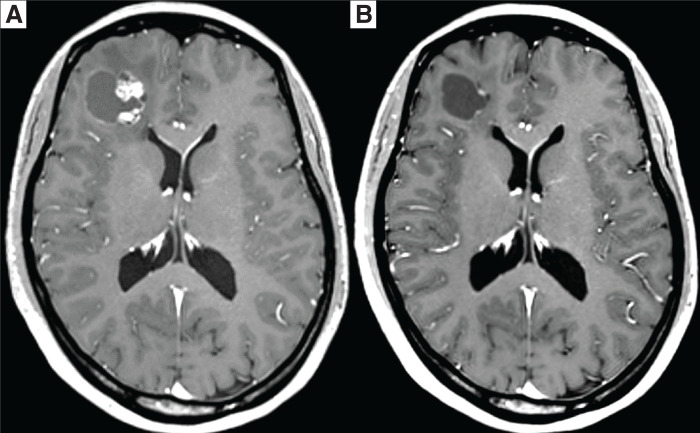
(A) Enhanced brain MRI (T1-weighted imaging) revealed an abnormal signal area with peripheral enhancement in the right frontal lobe, consistent with recurrent brain metastasis. (B) One month after reinitiation of alectinib, the metastatic lesion shrank markedly, and contrast enhancement was reduced on follow-up MRI.
